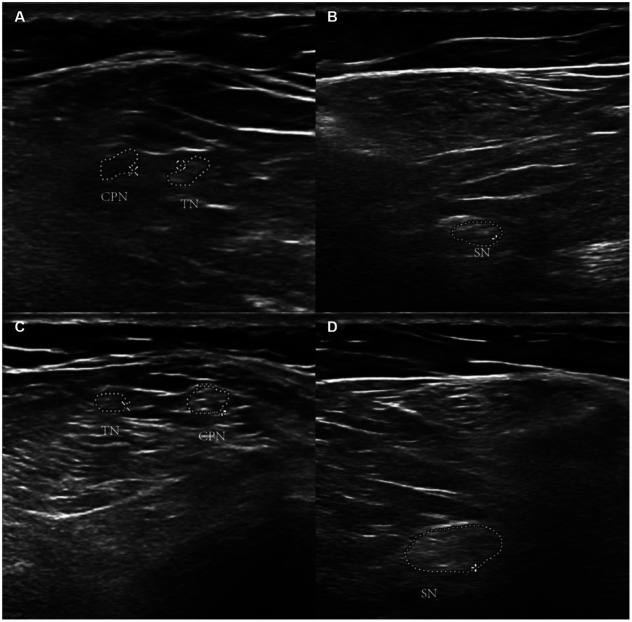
Ultrasonographic image of nitrous oxide-Induced peripheral neuropathy. Panels (A,B) show the echo enhancement around peripheral nerves in the left lower limb. Panels (C,D) are the right lower limb. ^*^TN refers to the tibial nerve. CPN refers to common the peroneal nerve. SN refers to the sciatic nerve.
